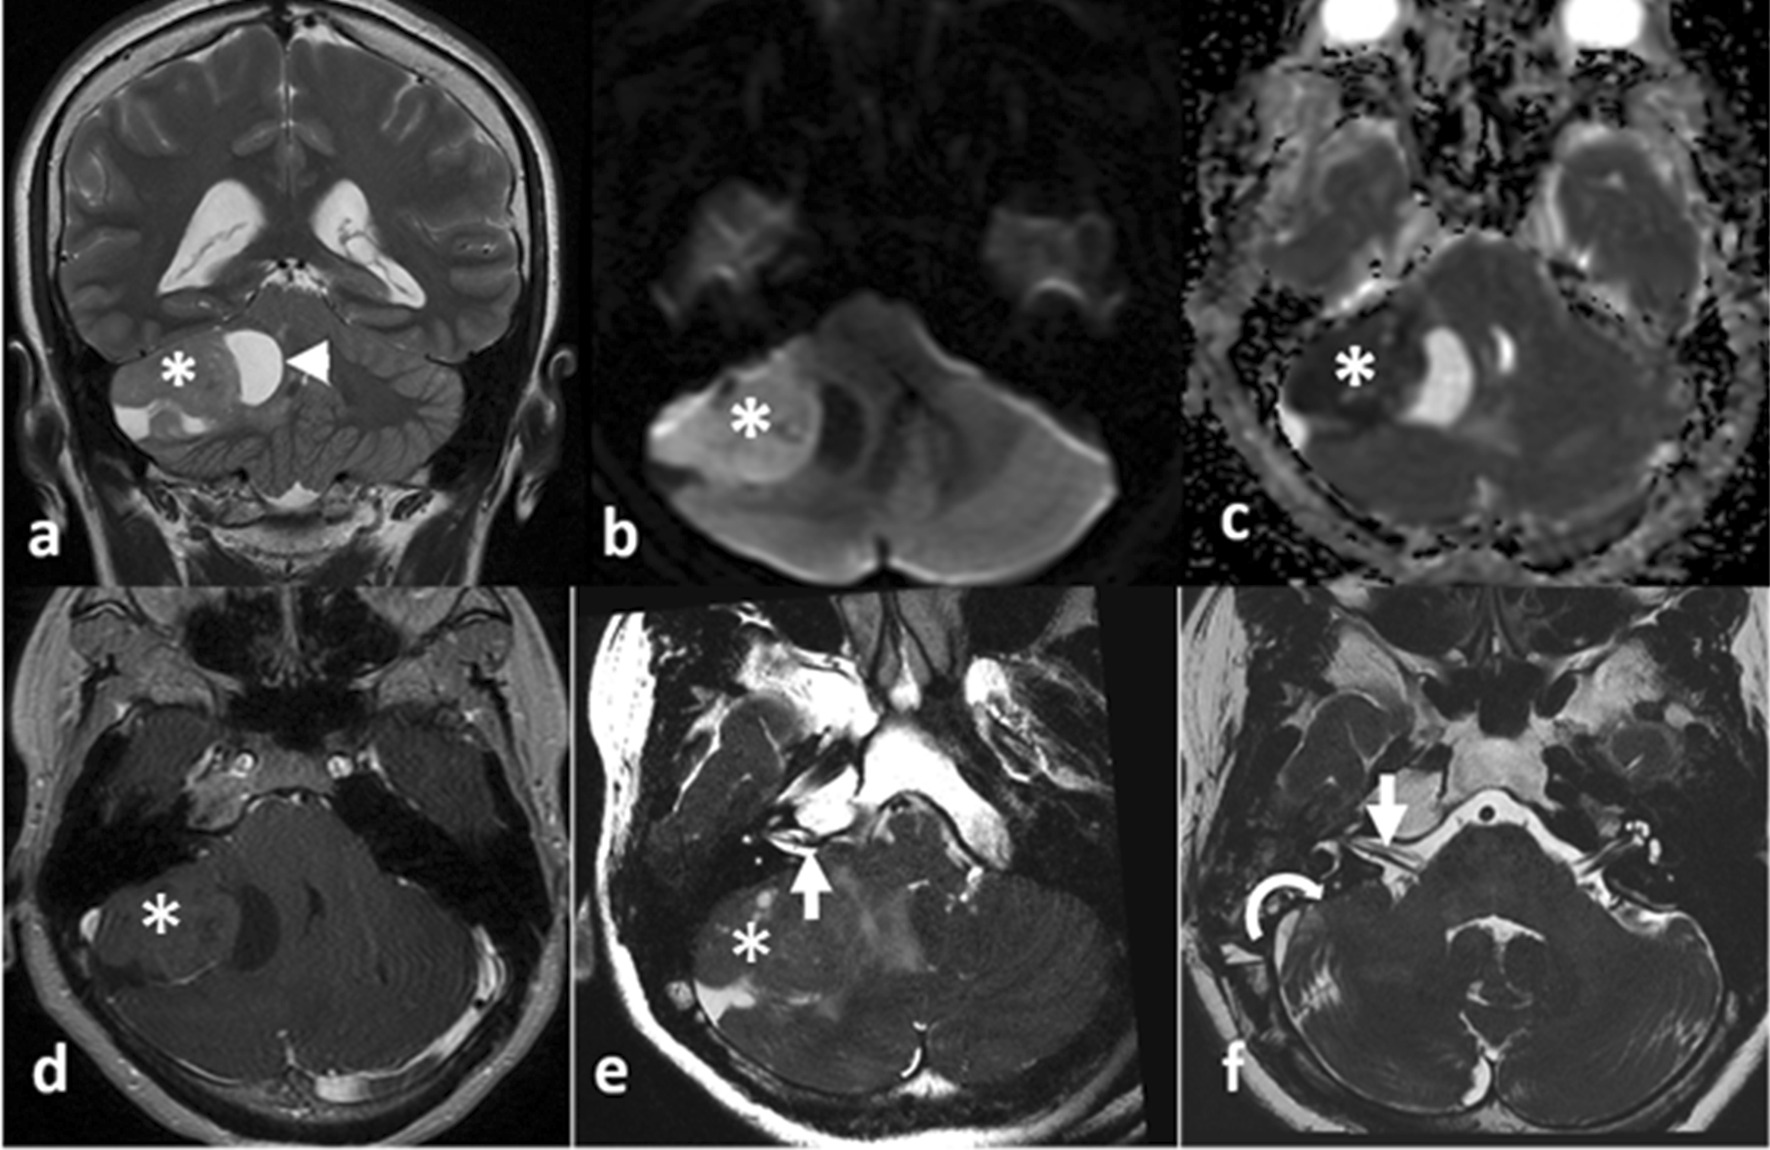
Magnetic resonance imaging from the preoperative examination (a–e) and 3 weeks after the operation (f). A mixed-signal mass (asterisk) was observed in the right cerebellopontine angle, presenting with solid and cystic components (white arrowhead) on T2-weighted coronal imaging (a). The solid component showed hypersignal on diffusion-weighted imaging (b) and significant hyposignal on the apparent diffusion coefficient map (c). After contrast injection, this mass was poorly enhanced (d). On the 3D-constructive interference in steady-state sequence (e), the VII/VIII cranial nerve complex (white arrow) was observed to be anteriorly displaced. The postoperative axial 3D-constructive interference in steady-state sequence (f) revealed a normal right cerebellar hemisphere (white curved arrow), and the VII/VIII cranial nerve complex (white arrow) returned to its expected location
